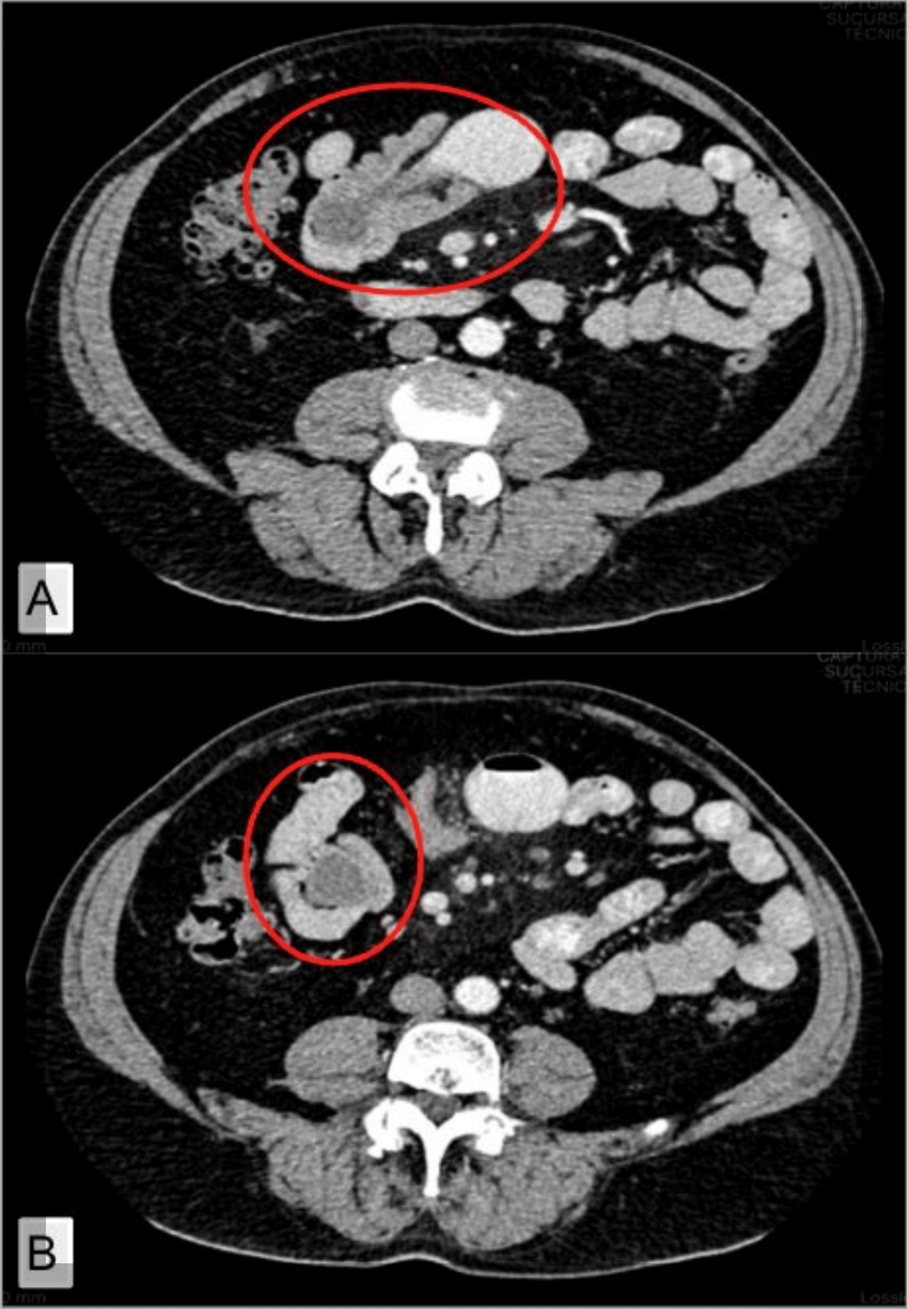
Computed tomography of the abdomen and pelvis with IV contrast A polypoid lesion of the distal jejunum that causes small bowel intussusception, considered the first possibility of a gastrointestinal stromal tumor. A) Red circle - sausage or sandwich sign. B) Red circle - target or “bullseye” sign.
